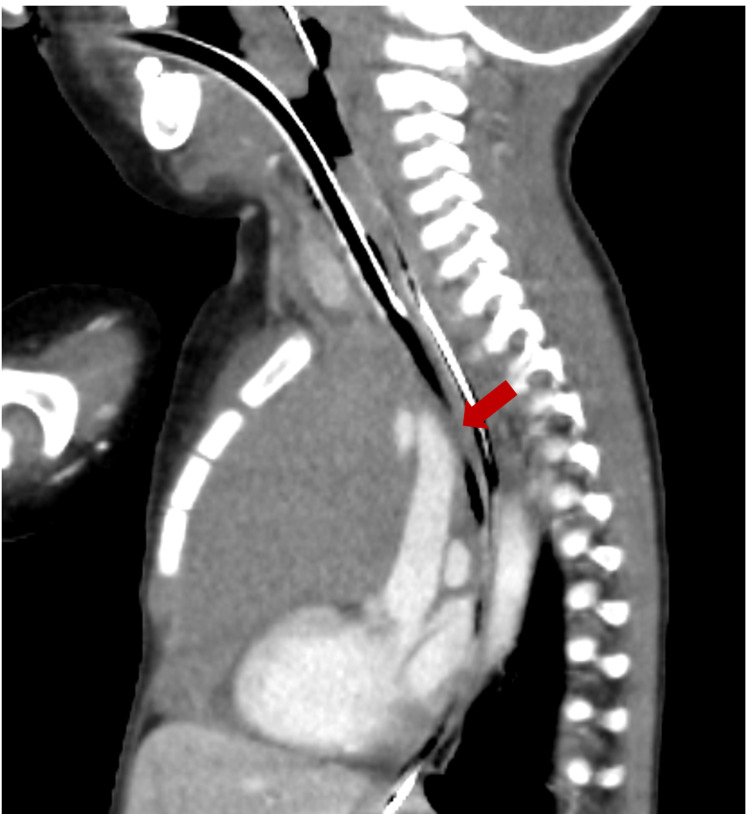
Contrast-enhanced CT The trachea distal to the intubation tube collapsed due to compression by the tumor.
